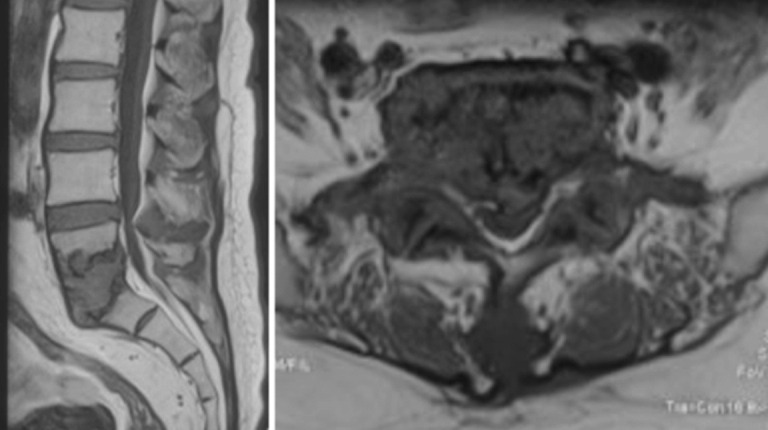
Magnetic resonance imaging (MRI) T1 weighted of sagittal and axial views which showed irregular inferior end plate L5 and superior end plate S1, expanded.
